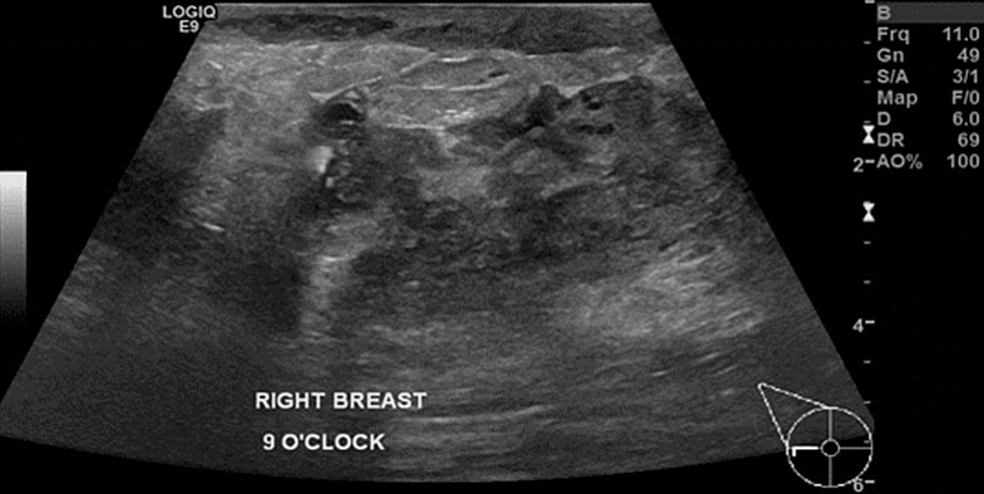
Right breast ultrasound showed heterogeneity with fluid collections more prominent at outer quadrant of right breast.
